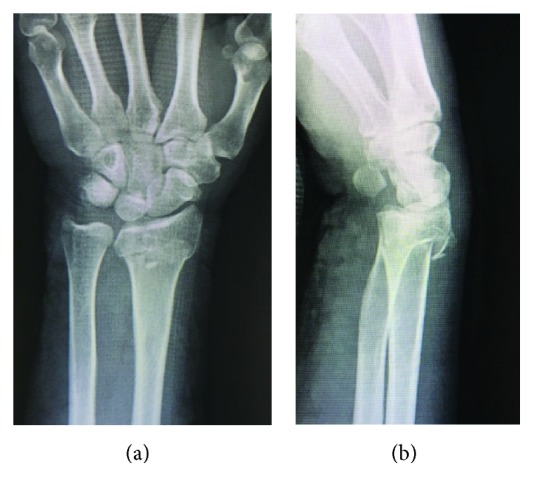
Plain X-ray films on her visit to our hospital. (a) Frontal plain X-ray image. (b) Lateral plain X-ray image. Plain X-ray images showed a distal radius fracture accompanied by dorsal displacement of the distal bone fragment. Due to the skin state, conservative treatment by external fixation, such as splinting and casting, was difficult. Surgery after improvement of the skin state was considered to be more invasive due to bone union. Therefore, osteosynthesis using a minimally invasive approach was planned.
